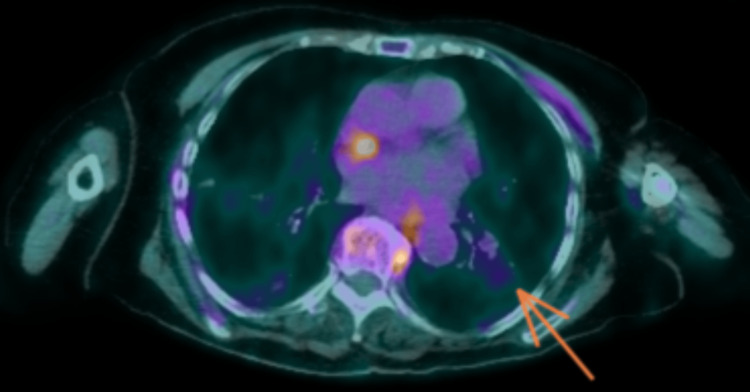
Axial section of fludeoxyglucose (18F) (FDG) positron emission tomography. The image demonstrates mild FDG activity of the thrombus in the lateral basal segmental branch of the left lower lobe (orange arrow).
